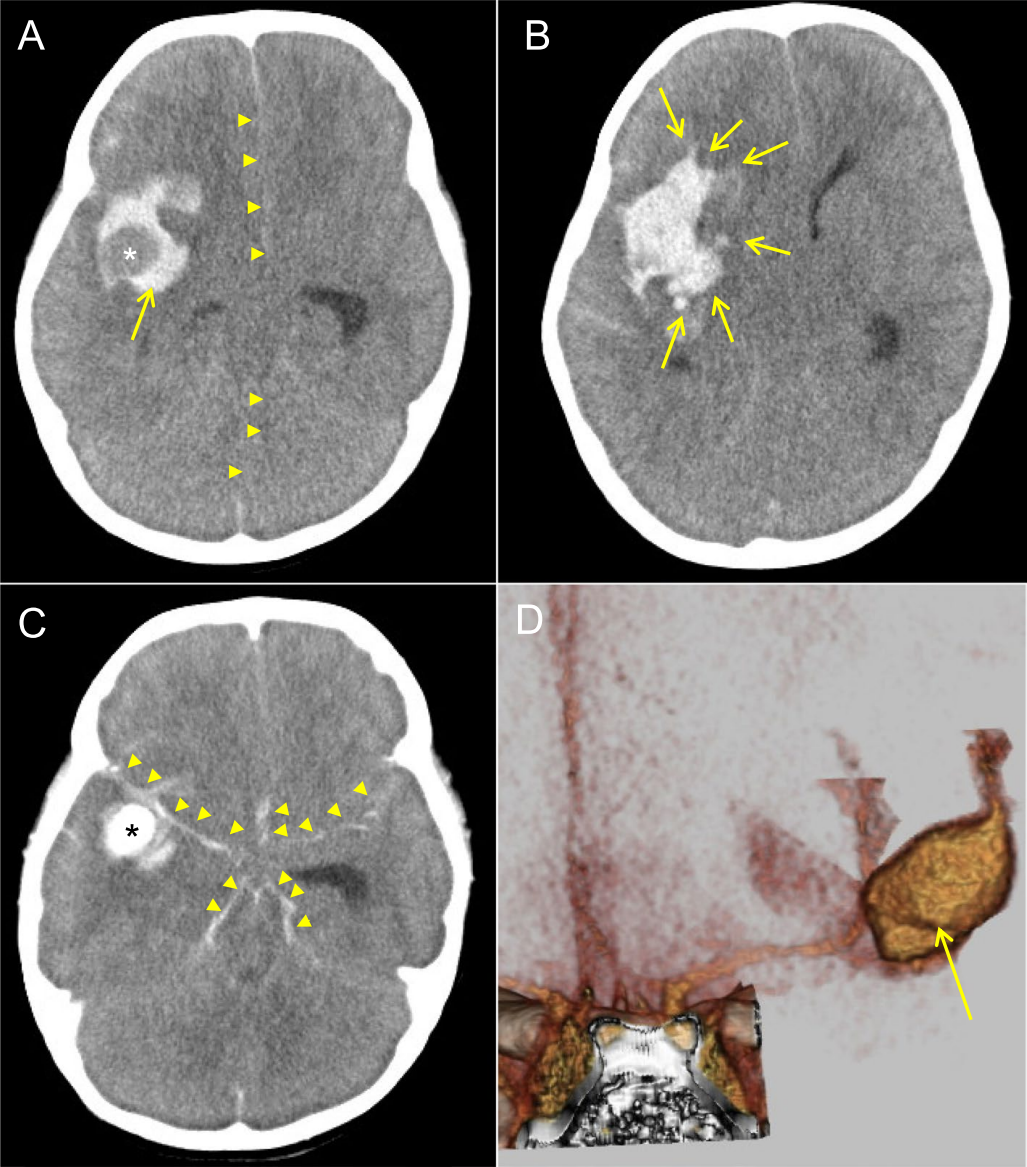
Plain and contrast-enhanced CT scans of the aneurysm in the present case. A A plain CT on admission in the present case. Arrow indicates the massive hemorrhage and the devoid of hemorrhagic signal (asterisk) in the right MCA region. Arrowheads indicate the prominent midline shift to the left hemisphere. B A plain CT on admission. The hemorrhagic lesion was extended to the surrounding parenchyma involving the caudate and lentiform nuclei (arrows). C A contrast CT shows the presence of an aneurysm located at the right MCA region (asterisk) and subarachnoid hemorrhage (arrowheads). D A stereographic reconstitution of the contrast head CT depicting the fusiform structure of MCA aneurysm (arrow: 21 × 13 × 12 mm in size)
